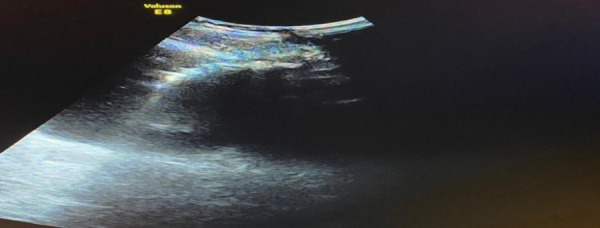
Ultrasound Pelvis showing hernia mesh projecting in urinary bladder
